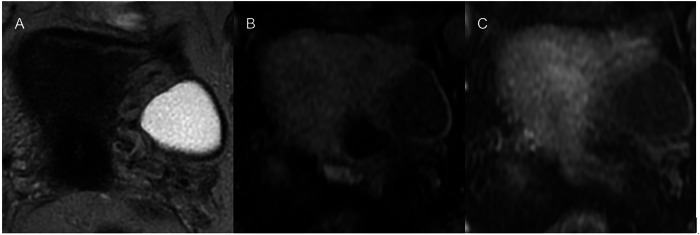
MRI in a 62-year-old patient following the detection of a cystic formation during transvaginal ultrasound. In the left adnex, an unilocular cyst measuring 34 mm with simple fluid content, hyperintense on T2 (A) and hypointense on T1 (B), with a thin wall and no enhancement on post-contrast image (C). Related to the dimensions and the postmenopausal status of the patient, the O-RADS MRI score 2 is assigned
